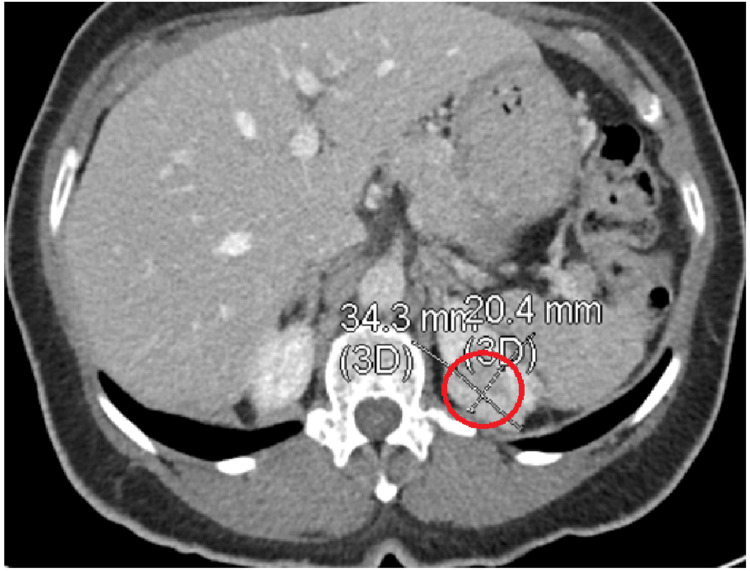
Computed tomography of the abdomen and pelvis with contrast The figure shows a poorly defined enhancing solid left superior renal upper pole with a posterior cortical mass about 34.3 mm × 20.4 mm in size, further highlighted by the red circle. This has extended beyond the renal capsule.
